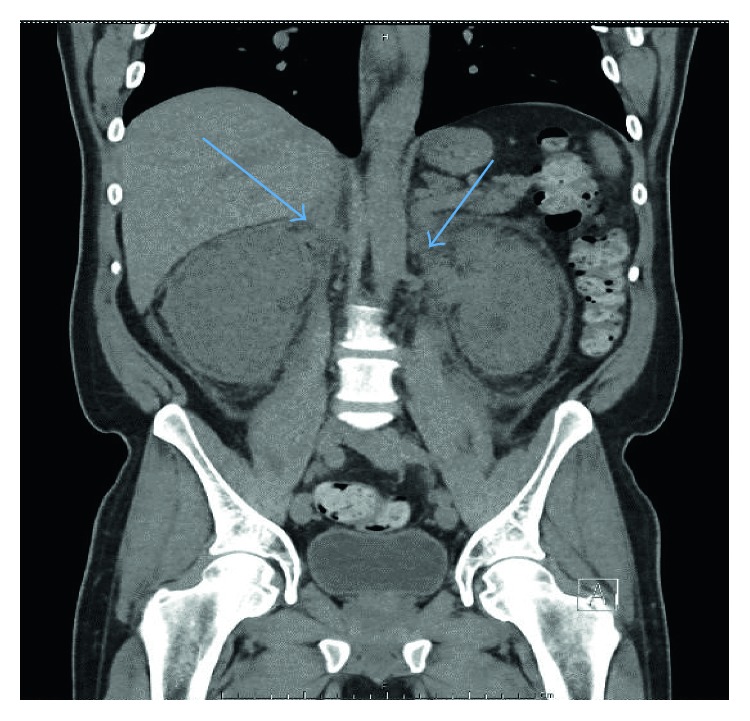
CT chest/abdomen/pelvis showing soft tissue encasing the adrenal glands, kidneys, infrarenal abdominal aorta, and soft tissue in the presacral space consistent with Erdheim-Chester disease.
